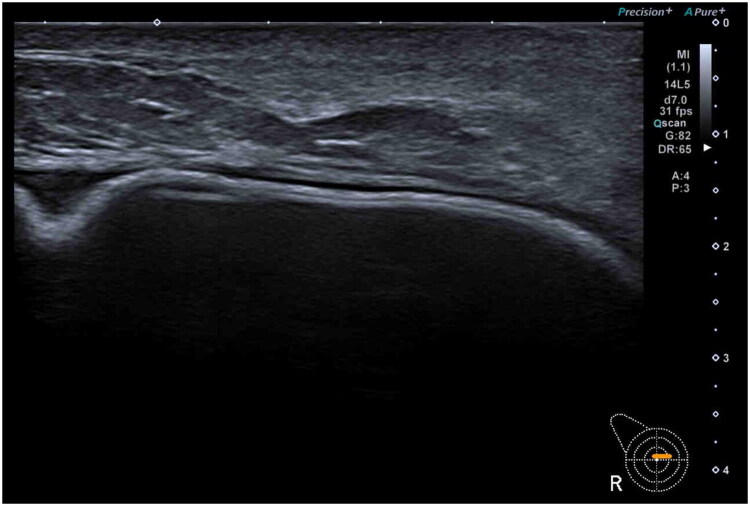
Right breast ultrasound showing a small seroma surrounding the implant. No organized abscess was identified.
